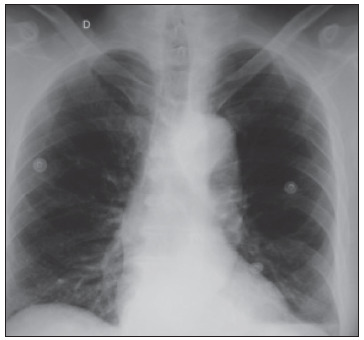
Chest X-ray showing enlargement of mediastinum.
